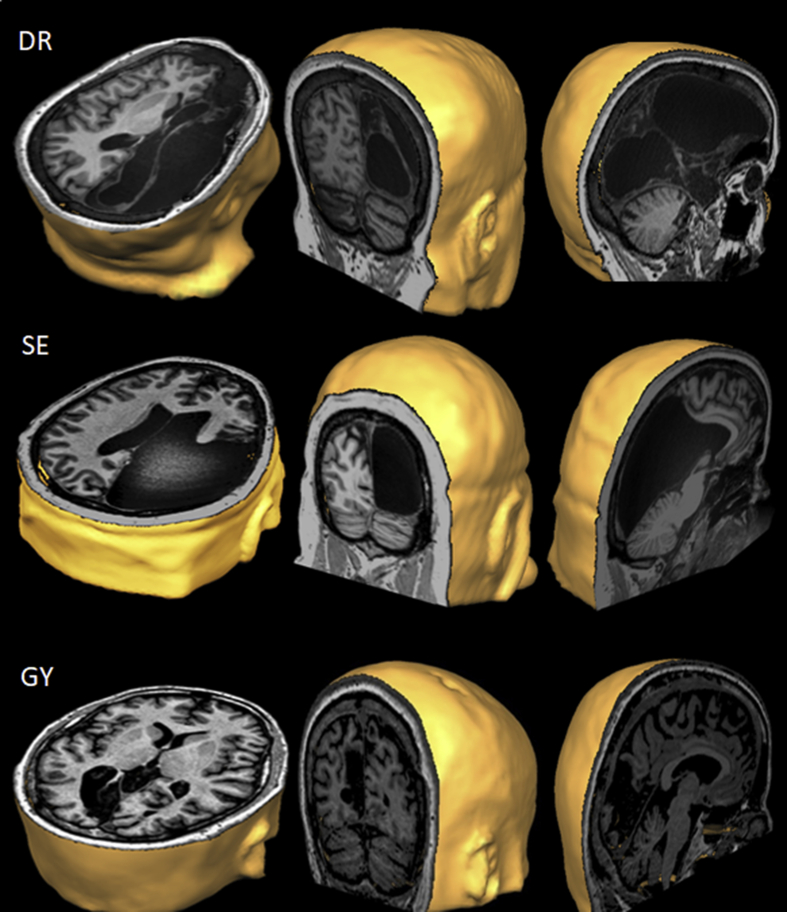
Sections of T1-weighted 3T MRI anatomical scans of DR (top), SE (middle), and GY (bottom) showing the lesion of the visual cortex in transverse (left), coronal (middle) and sagittal (right) views.
